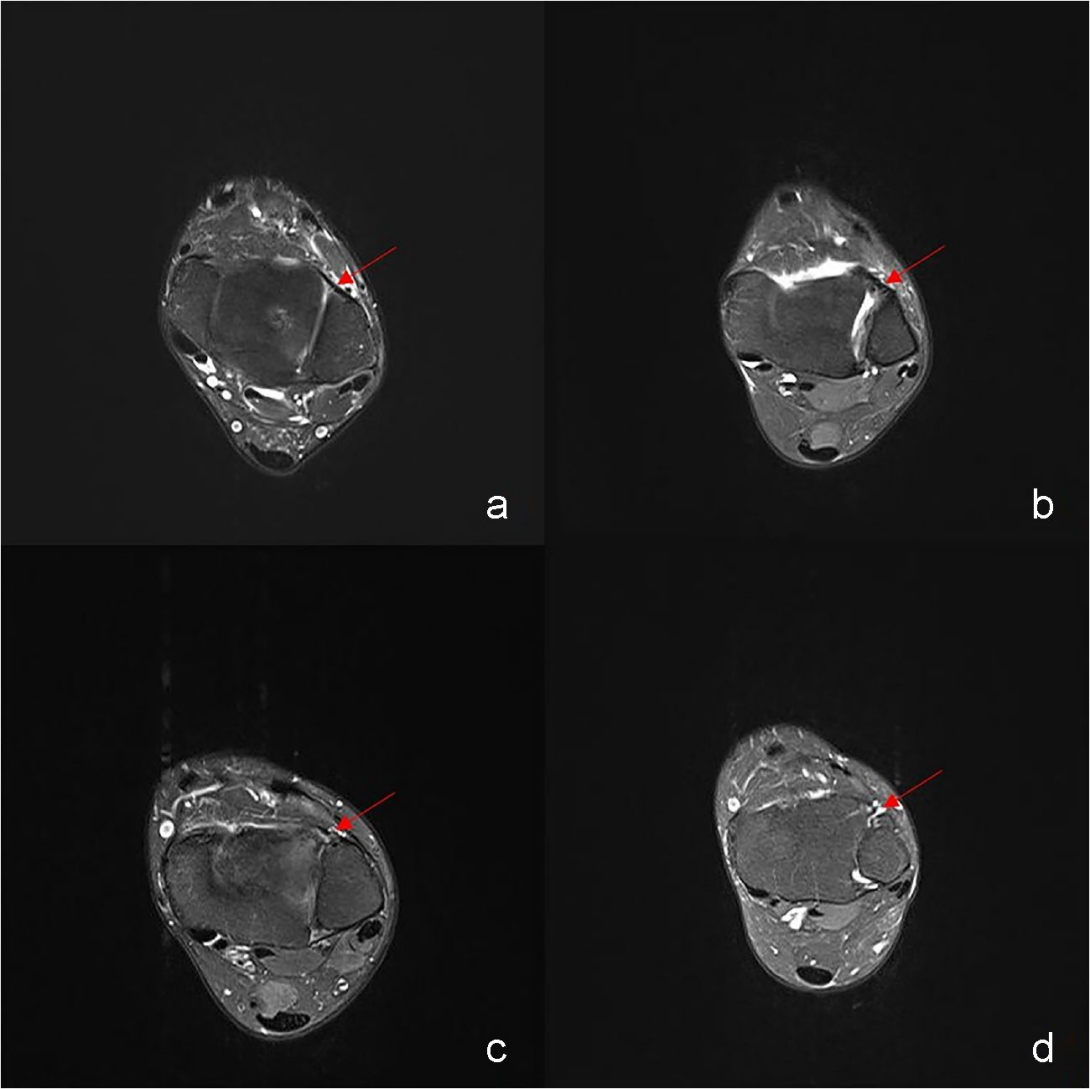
a–d Imaging findings of adjusted and non adjusted AITFLs on a route MRI protocol (T2 weighted images obstructed approximately at level of tidal flat on horizontal level/plane). When AITFL did not exhibit discontinuity or thickening, it was considered not adjusted (arrow in a, a score of 0). AITFL was seen as being adjusted when it was thick (arrow in b, a score of 1), with a wavy contour or discontinuity (arrow in c, a score of 2), or absent (arrow in d, a score of 3). AITFL, anterior inferior tibiofibular ligament
